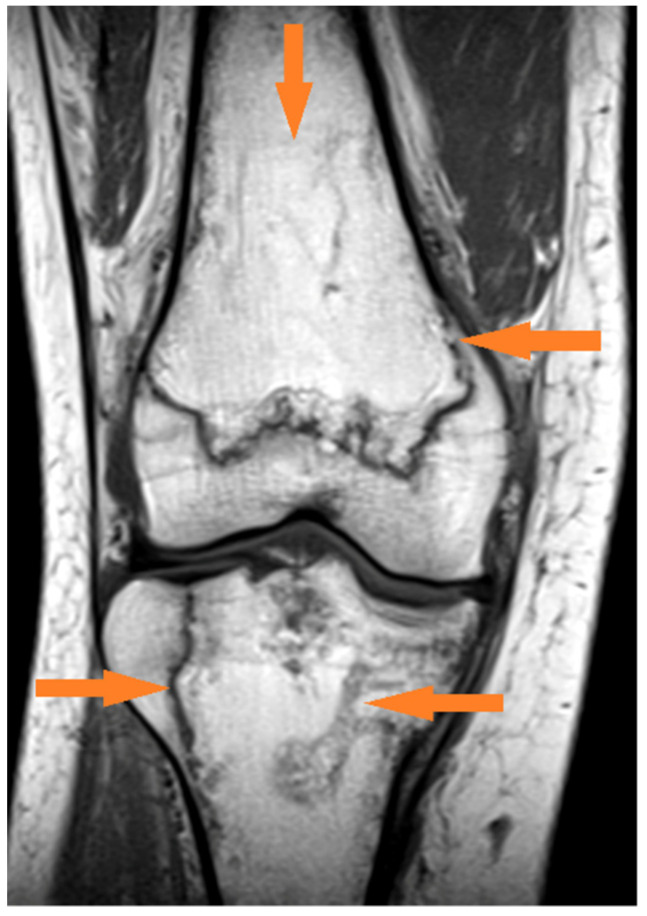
Changes found in the knee joint on T1-weighted sequence in a 43-year-old woman: geographical demarcation of bone infarction in the femur and tibia (orange arrows).
